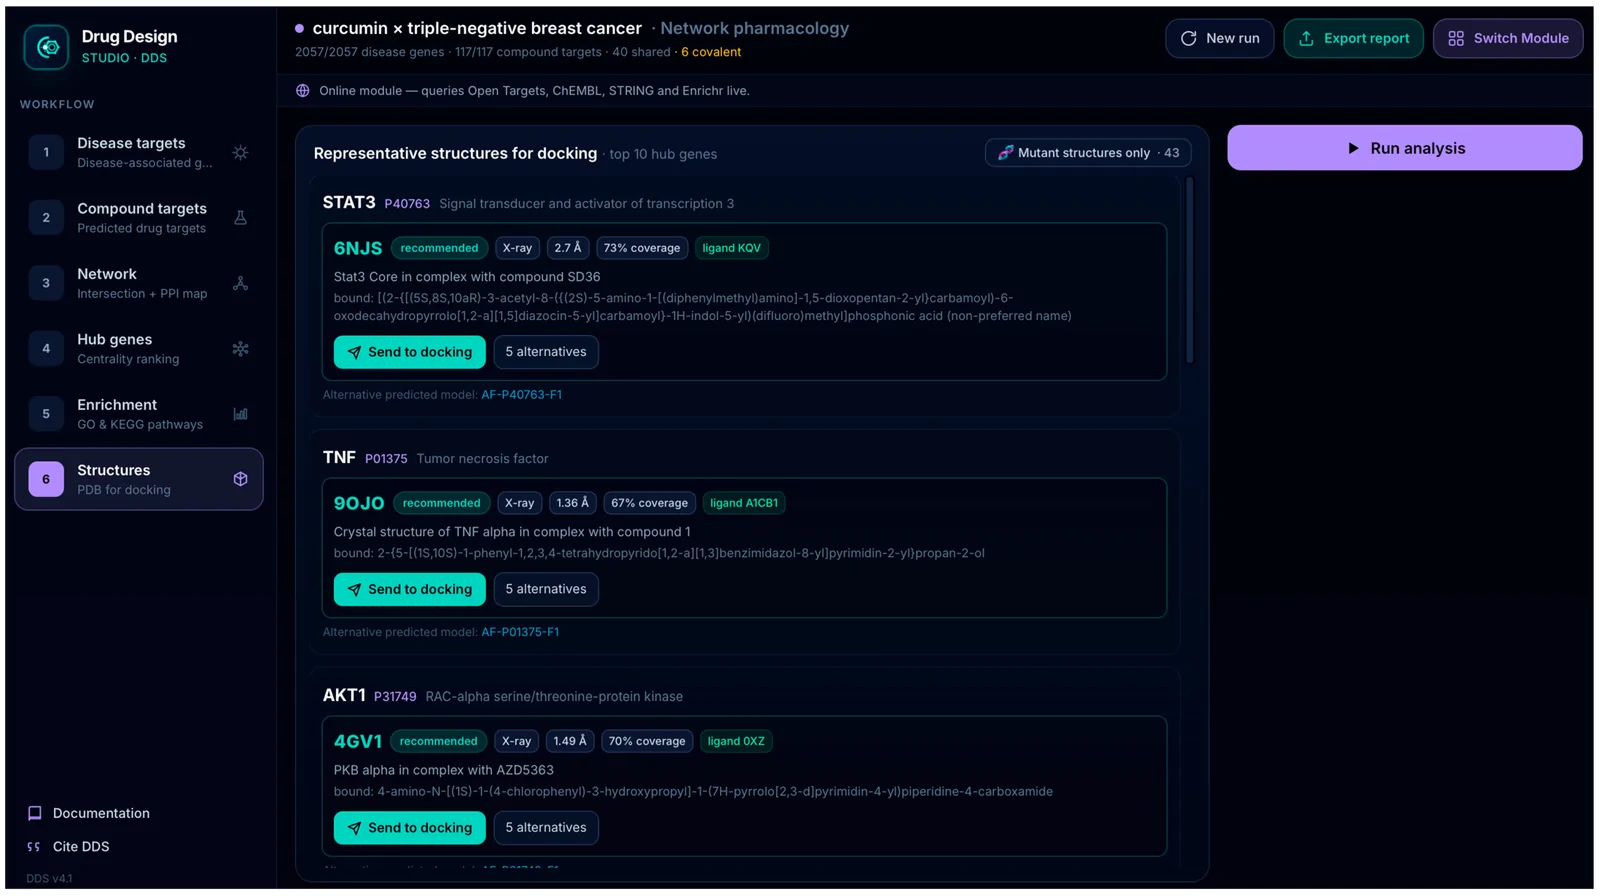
Representative-structure selection for docking in the DDS 2.0 Structures step, shown for the top 10 hub genes of the curcumin × triple-negative breast cancer network. For each hub gene, DDS resolves the gene to its reviewed human UniProt entry and ranks the available PDB structures for docking suitability by experimental method, resolution, domain coverage and bound ligand. Each card lists the recommended structure with its metadata badges (method, resolution, % domain coverage, bound ligand) and a short description, together with a one-click send to docking hand-off to the DDS docking module, a set of five ranked alternatives, and a link to the corresponding AlphaFold model shown beneath the card (e.g., AF-P40763-F1 for STAT3). A “Mutant structures only” filter—here surfacing 43 experimentally resolved mutant structures across the hub genes—additionally restricts the selection to mutant forms of the targets (Section 3.11). Representative examples: STAT3 → 6NJS (X-ray, 2.7 Å, 73% coverage, ligand KQV); TNF → 9OJO (X-ray, 1.36 Å, 67% coverage, ligand A1CB1); and AKT1 → 4GV1 (X-ray, 1.49 Å, 70% coverage, ligand 0XZ). For hub genes lacking a suitable experimental structure, DDS instead offers the corresponding AlphaFold model as the docking starting point, ensuring that even difficult targets have a defined structure.
